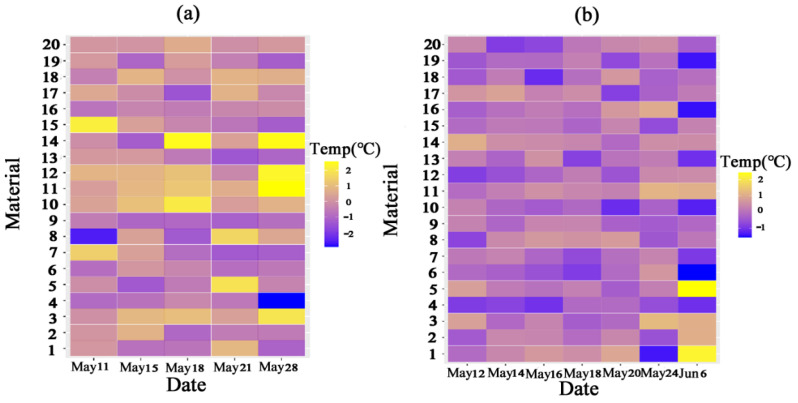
Normalized trend of CT data of wheat materials in 2022 (a) and 2023 (b). The Y-axis represents the 20 materials, and the X-axis represents the time at which the CT was measured.
